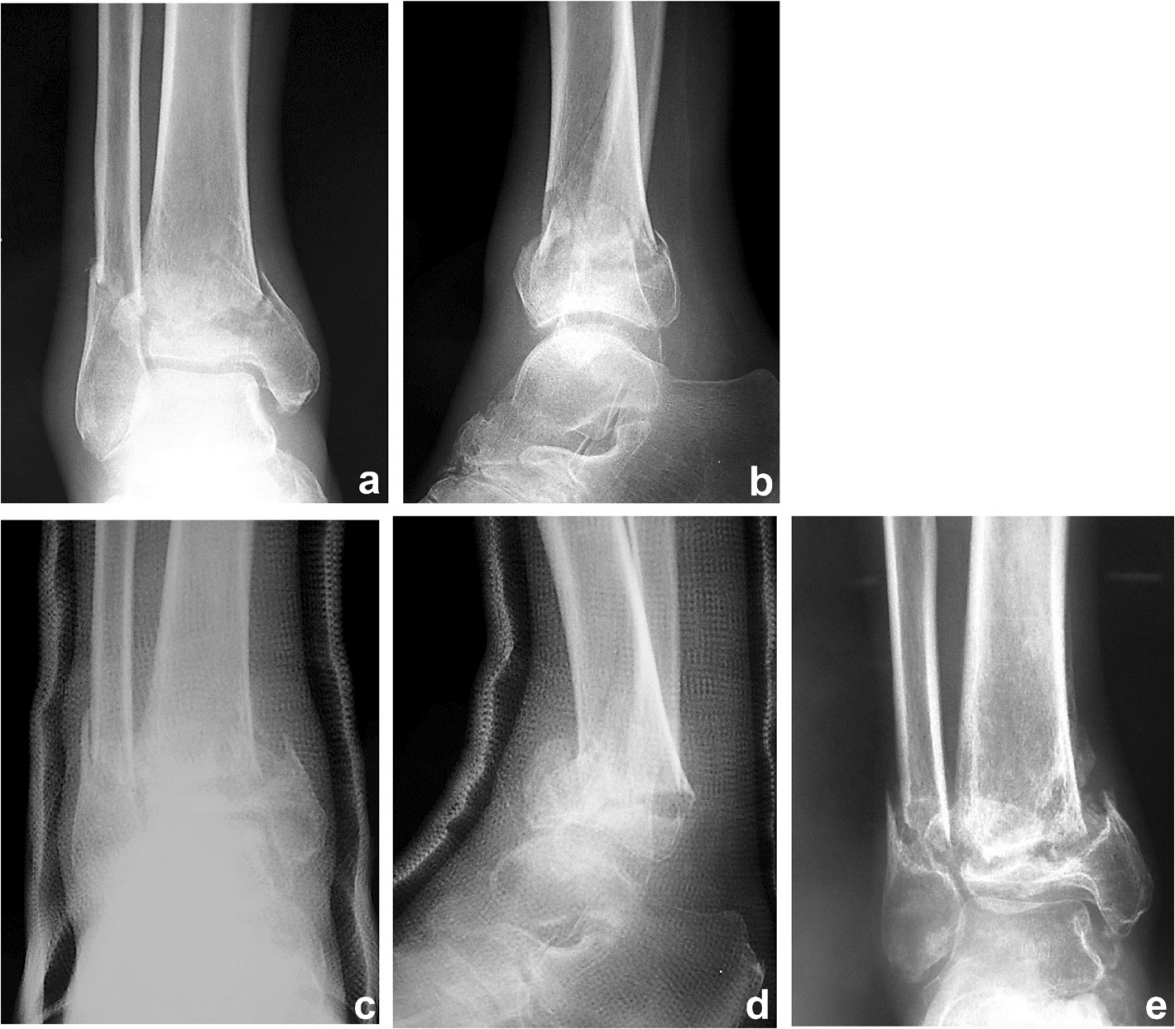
Preoperative radiographs. (a, b) The initial plain X-ray showed a right ankle fracture (AO type 43-A1.3). (c, d) Radiographs 1.5 months after the injury. (e) Radiograph of nonunion 3 months after injury on initial consultation.
